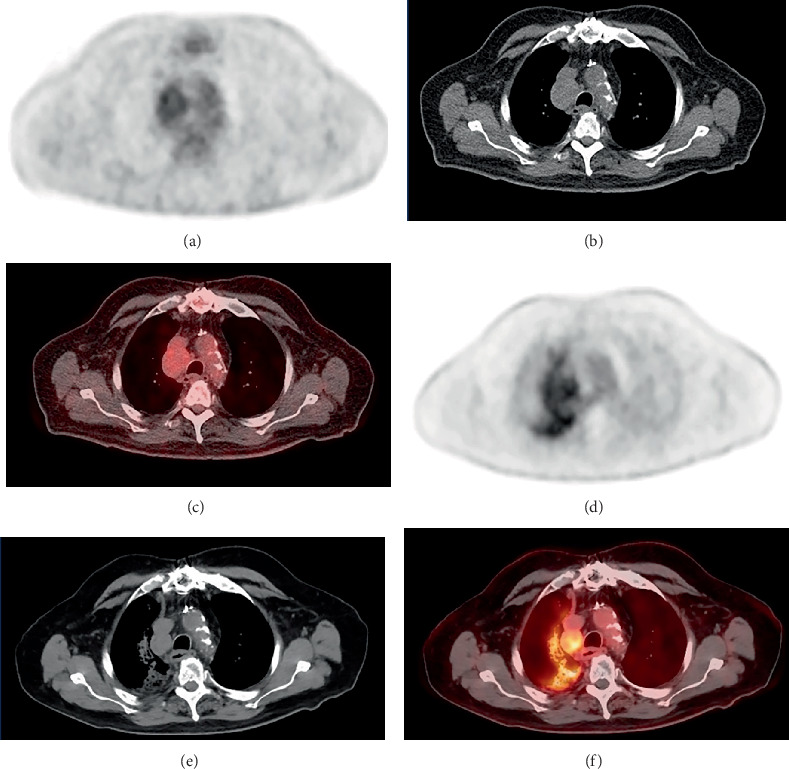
Restaging after nephrectomy showed stable FDG uptake by the mediastinal metastasis as demonstrated on axial PET/CT images (a–c). The patient underwent further treatment with SBRT. Follow-up PSMA PET/CT (d–f) was obtained to restage prostate cancer approximately 9 months after the initial study which showed the pelvic prostate cancer metastasis and incidentally identified the renal mass. The decrease in size from 3.5 to 2.7 cm as well as the decrease in PSMA uptake (SUVmax 4.8, previously 8.4) associated with the right paratracheal ccRCC metastasis suggested a partial response to therapy, although the precise implications of changes in PSMA uptake warrant further investigation.
